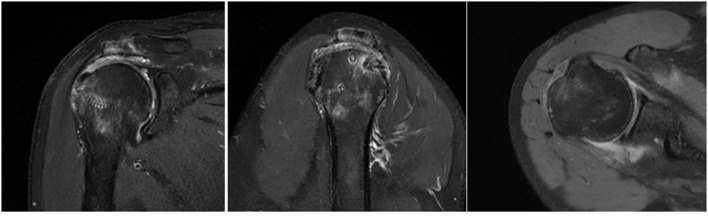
Sugaya's classification type III in postoperative MRI.
